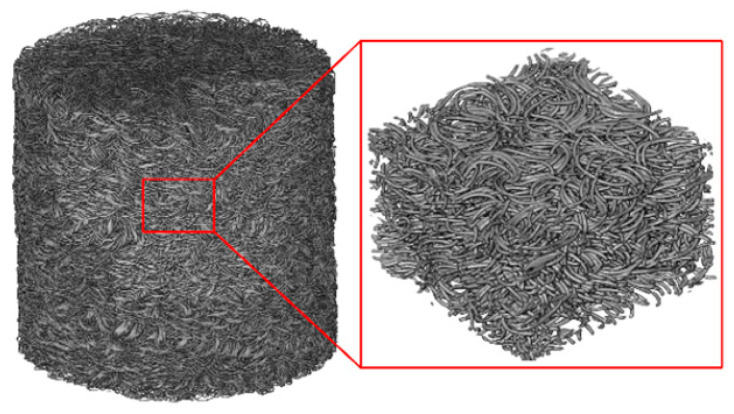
The meso-scale structure of an EMWM obtained by CT scanning.
